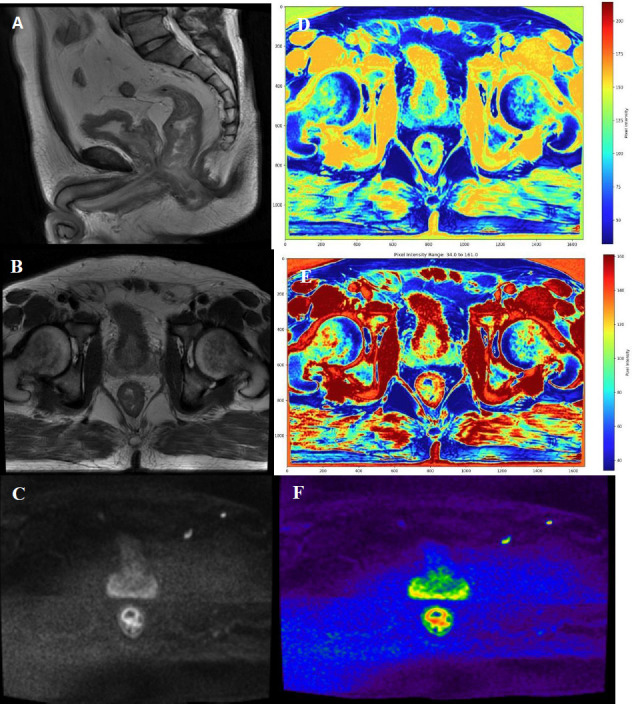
Colormap representation of MRI in a 56-year-old male with T2N1 stage rectal cancer: (A) Sagittal T2WI showing a tumor in the low rectum; (B) Oblique axial T2WI highlighting the rectal tumor; (C) Oblique axial DWI of the same tumor; (D) Default colormap representation of the tumor on an axial T2WI; (E) Alternative colormap representation of the tumor on axial T2WI; (F) Rainbow colormap representation of the tumor on axial DWI. This image serves as a visual representation; however, radiomic feature extraction was conducted using the original grayscale MRI data.
